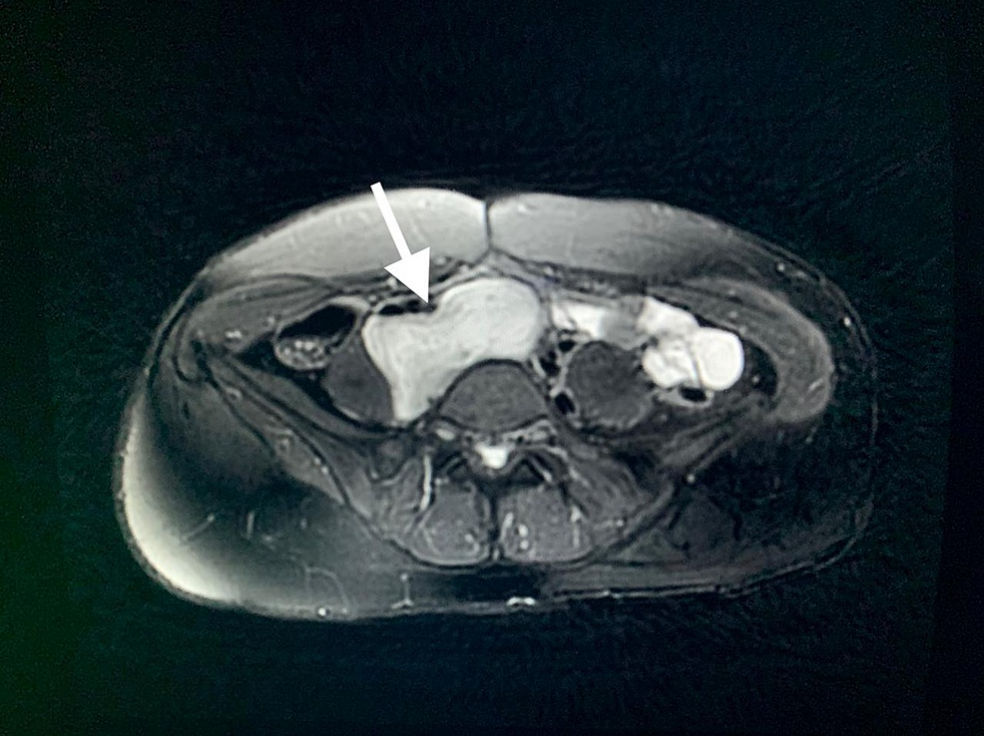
Abdominal MRI T2 featuring the high T2 with streaks of low T2 signal of the mass (pointed with a white arrow).
